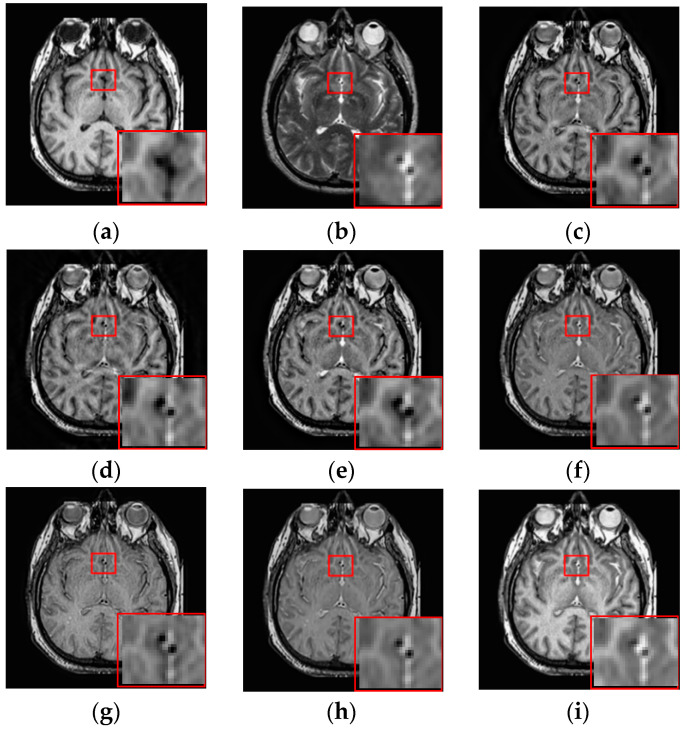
Fused results of MRI/CT medical images by different methods. (a) MRI; (b) CT; (c) DCTWT; (d) Curvelet; (e) NSCT; (f) Liu-ASR; (g) Kim; (h) Zhu; (i) Proposed.
